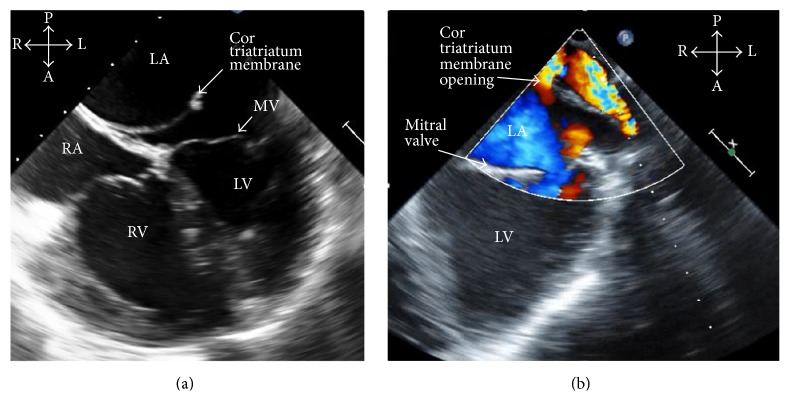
Transesophageal echocardiogram (a) is a midesophageal four-chamber view demonstrating cor triatriatum with a membrane separating the pulmonary veins from the left ventricle inflow and (b) is a midesophageal two-chamber view with Doppler flow demonstrating restrictive flow through the opening of the cor triatriatum membrane. LV: left ventricle, RV: right ventricle, LA: left atrium, RA: right atrium, MV: mitral valve, S: superior, I; inferior, R: right, L: left, A: anterior, and P: posterior.
